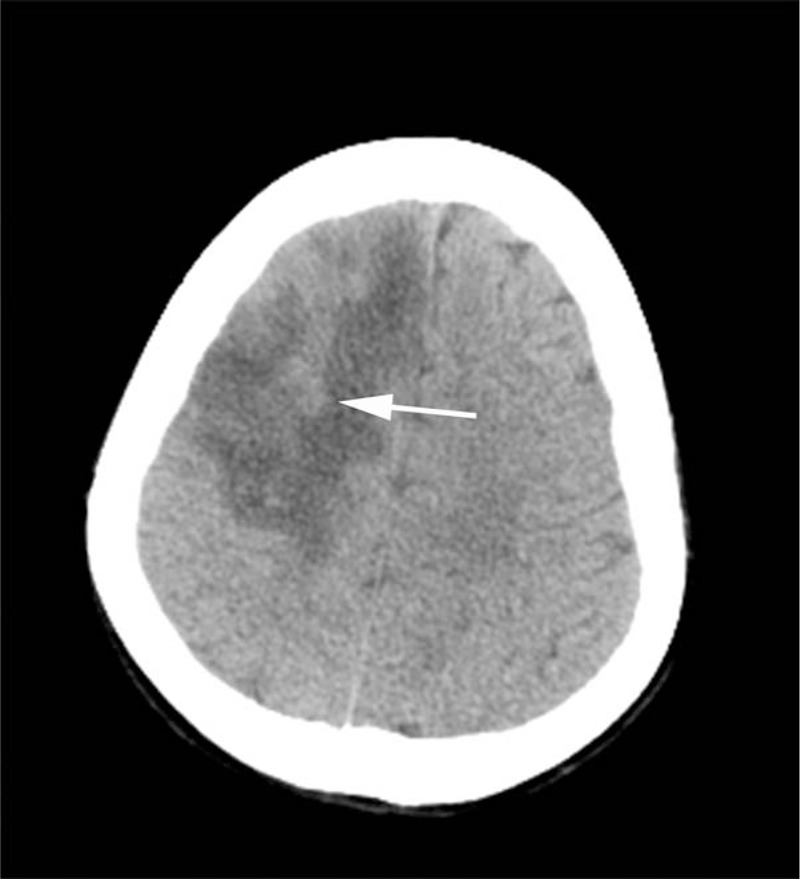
Brain CT at the 10-day follow-up scan shows a massive cerebral infarction in the left frontal lobe. CT = computed tomography.
